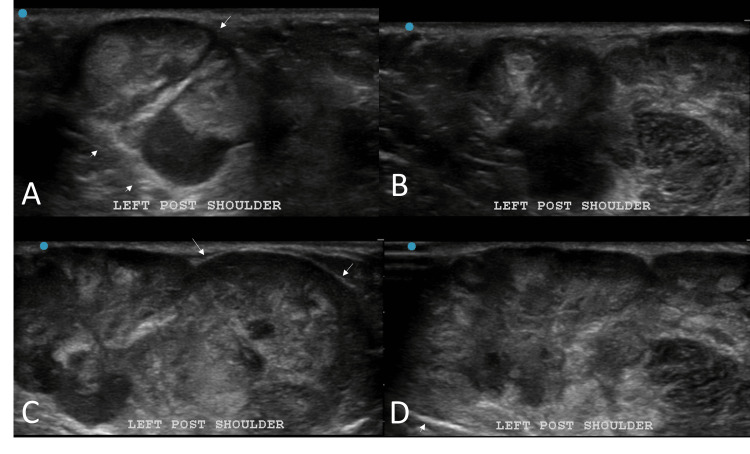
(A-D) Point-of-care ultrasound revealed a large (7.0 x 4.0 x 3.5 cm) encapsulated (arrows) subcutaneous mass with mixed hypoechoic and hyperechoic regions.
